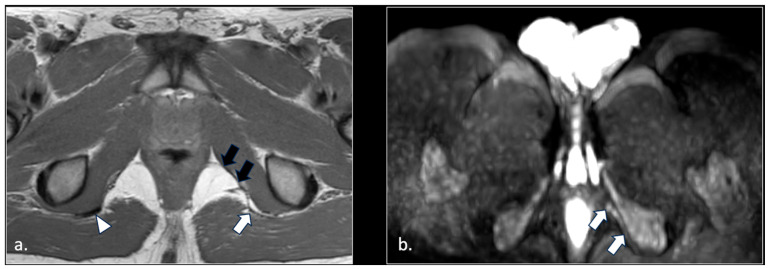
A 33-year-old man suffered from disabling perineal and scrotal pain lasting 3 years. The patient had been an amateur cyclist active for about 10 years. Palpation along the course of the left pudendal nerve, at the Alcock canal and medial to the ischial spine, reproduced the patient’s pain, consistent with the Valleix phenomenon [21]. MRI showed in an axial T1-weighted scan (a) a thinning of the left sacrotuberous ligament (white arrow), likely due to chronic microtrauma from cycling. Note the normal right sacro-tuberous ligament (arrowhead). Moreover, a thickening of the medial wall of the left Alcolck’s canal can be seen (double black arrows). The left pudendal nerve in the Alcock canal was hyperintense (arrows) on the b600 diffusion-weighted scan (b). A block of the left pudendal nerve was performed, with temporary relief (1 week) of symptoms.
